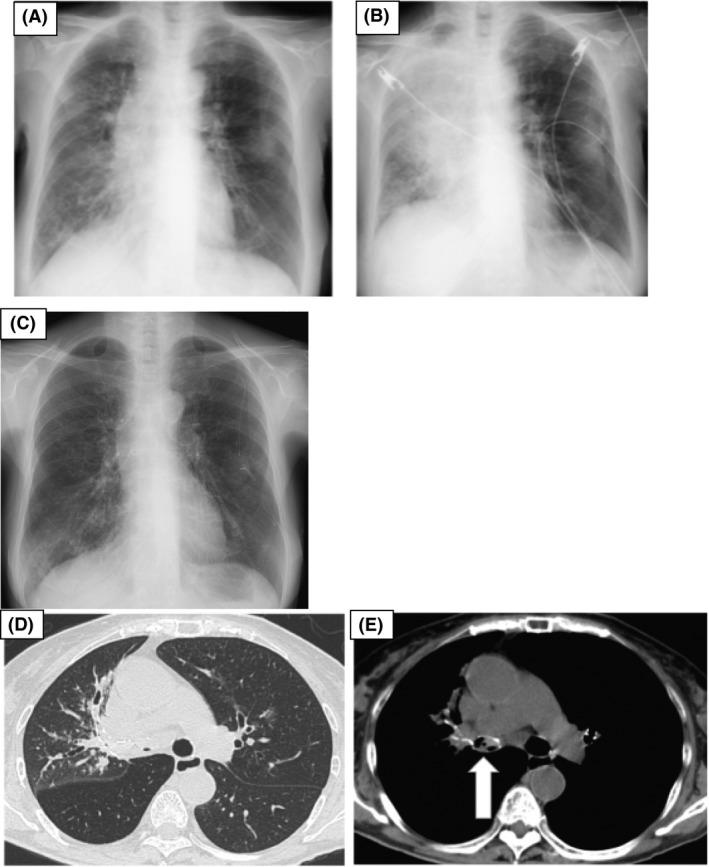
(A) Chest X‐ray on admission showed a mediastinal shift to the right for atelectasis, a reticulonodular pattern on the right lower lobe, and nodular density on the left middle lobe. (B) Ten hours after admission, permeability of the right lung was reduced due to massive atelectasis on the right lung field. (C) Seven days after admission, the atelectasis improved. (D, E) Computed tomography showed mucoid impaction and thickened bronchial walls in the right main bronchus, bronchodilation in the right middle lobar bronchus (arrow), and a centrilobular reticulonodular shadow in the distal lung field.
